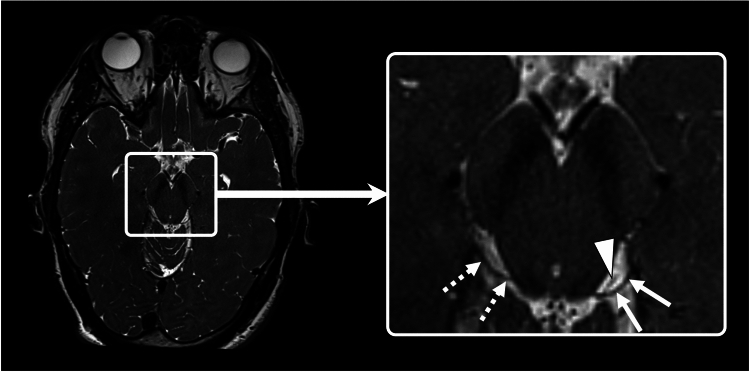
Visualization of the left (solid arrows) and right (dotted arrows) trochlear nerve using constructive interference in steady state (CISS) MRI in a healthy subject. Note how the trochlear nerve exits at the dorsal side of the brainstem. Due to the intensive vasculature of the brainstem, blood vessels are likely to be observable in close proximity to the trochlear nerve (arrowhead); proximity alone does not constitute a functionally relevant nerve compression syndrome
